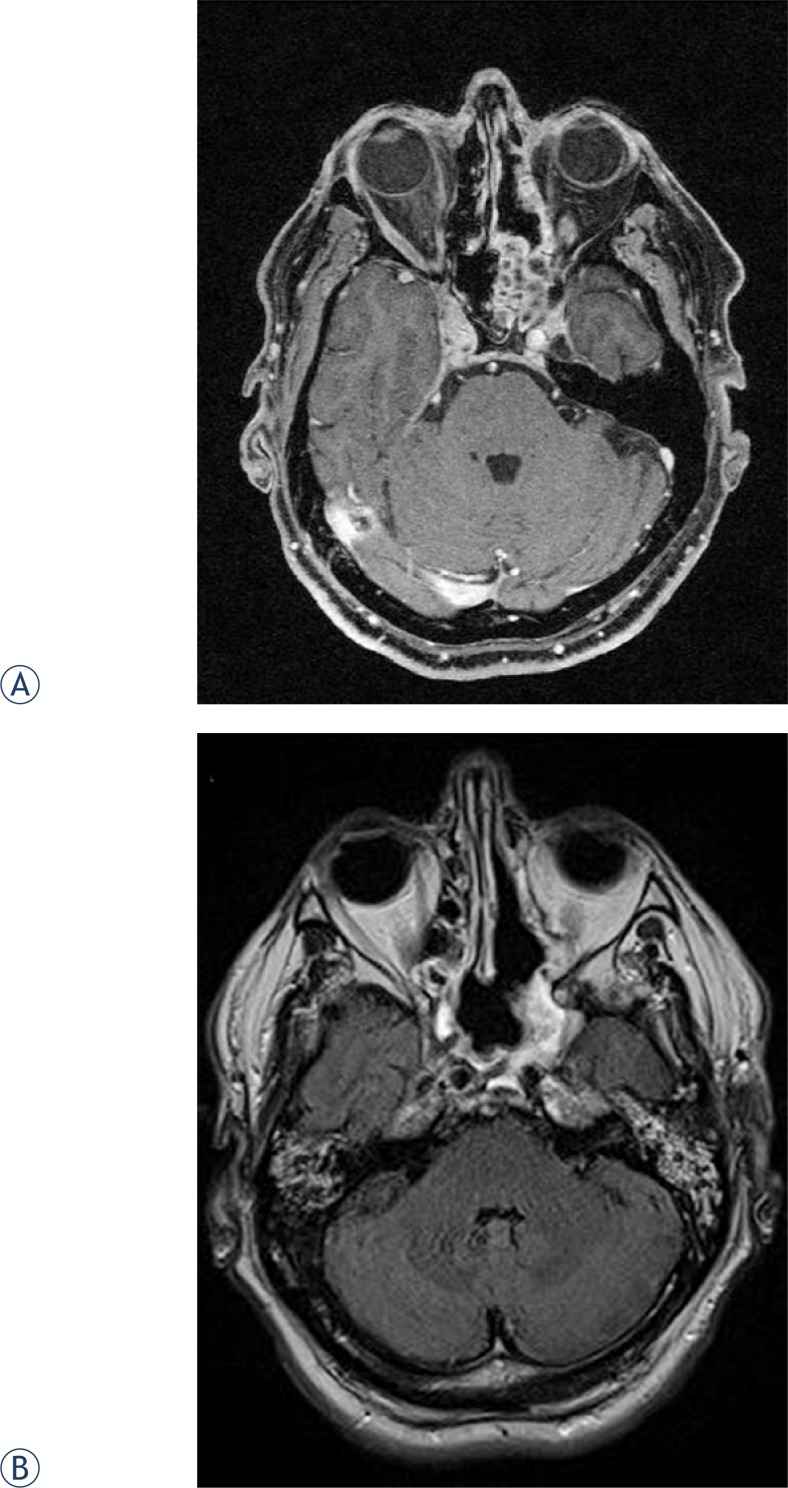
A. Six months after radiotherapy (post-contrast MRI T1 GE WI with fat suppression). B. Three years after diagnosis (post-contrast MRI T1 SE WI with fat suppression).
